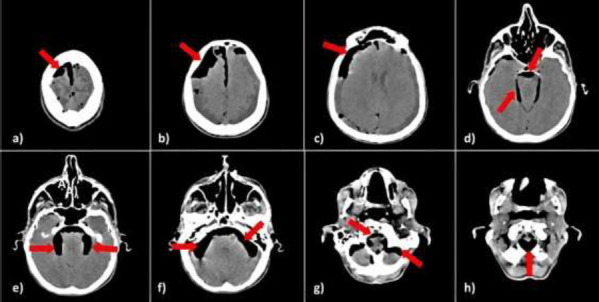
Bilateral pneumocephalus and air density in the foramen magnum observed in cranial CT scans. A subdural effusion could be seen in all sections. This finding supports the diagnosis of post-surgical pneumocephalus.
